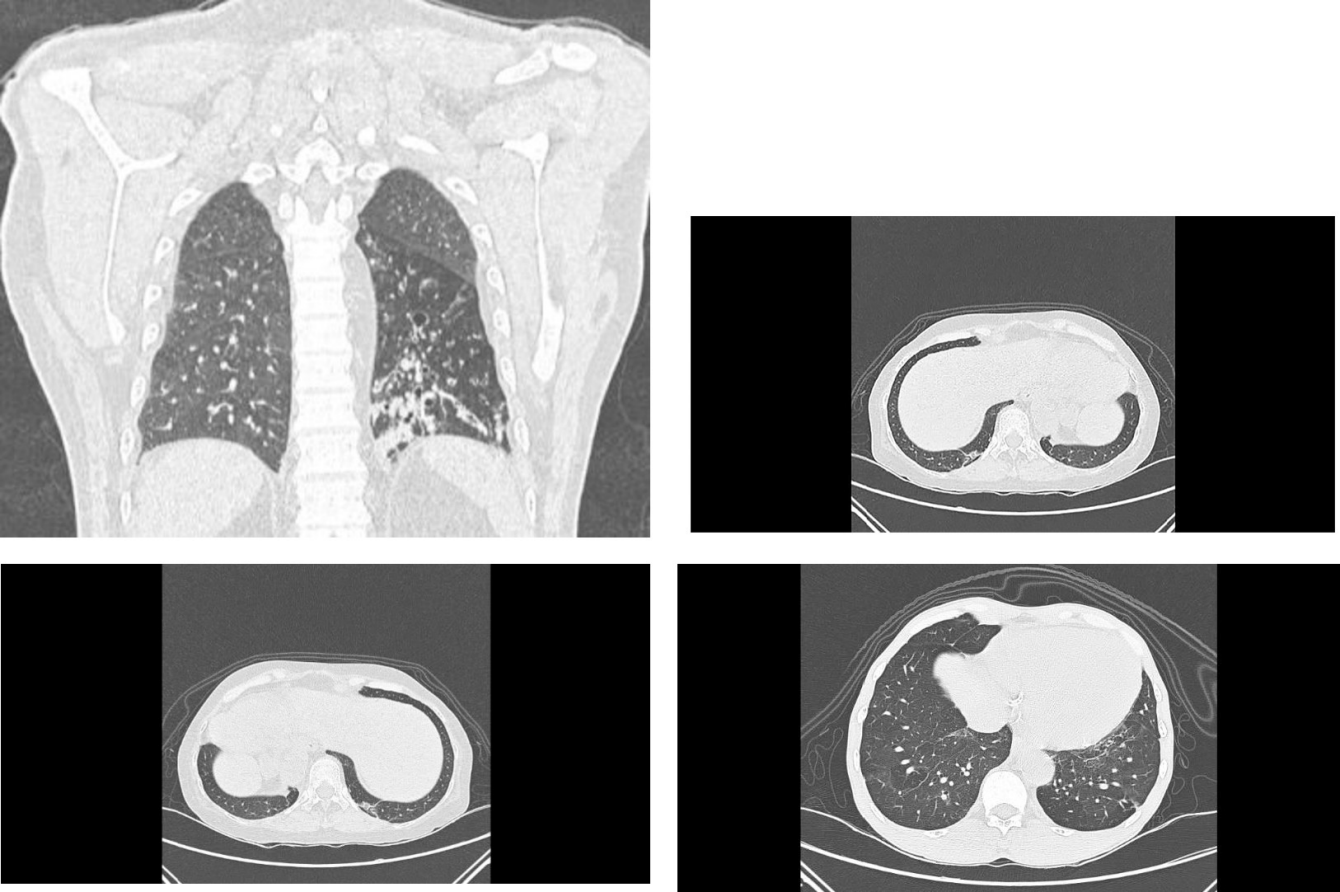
Radiological changes after COVID-19. A: Coronal (A) and axial (B) computed tomography (CT) in lung window showing evidence of bronchiectasis at basal segment of left lower lobe. B: Axial computed tomography (CT) in lung window showing evidence of ground-glass opacity at posterior aspect of both lower lungs. C: Axial computed tomography (CT) in lung window showing band-like parenchymal opacity at posterior aspect of right basal lung. D: Axial computed tomography (CT) in lung window showing reticulation at both basal lung.
